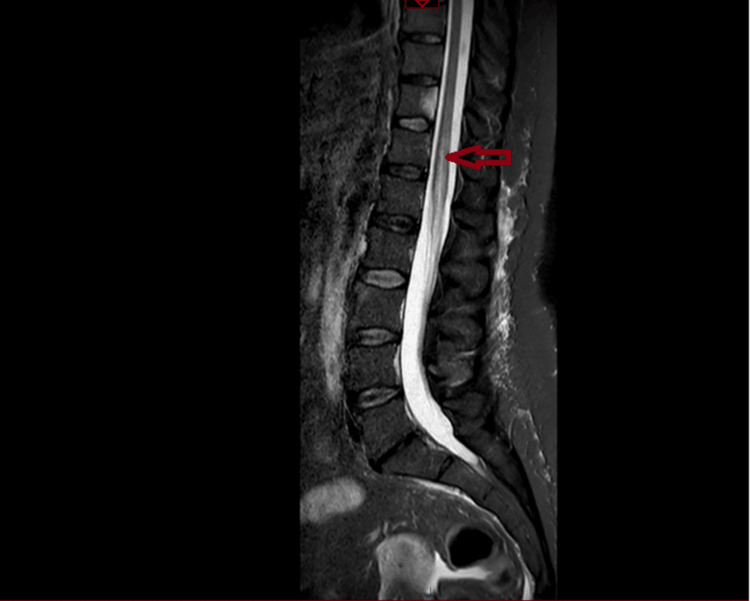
Sagittal STIR MRI of the thoracolumbar spine demonstrating linear intramedullary hyperintensity extending from the conus medullaris to approximately the T12 superior endplate, consistent with acute spinal cord infarction STIR: short tau inversion recovery
